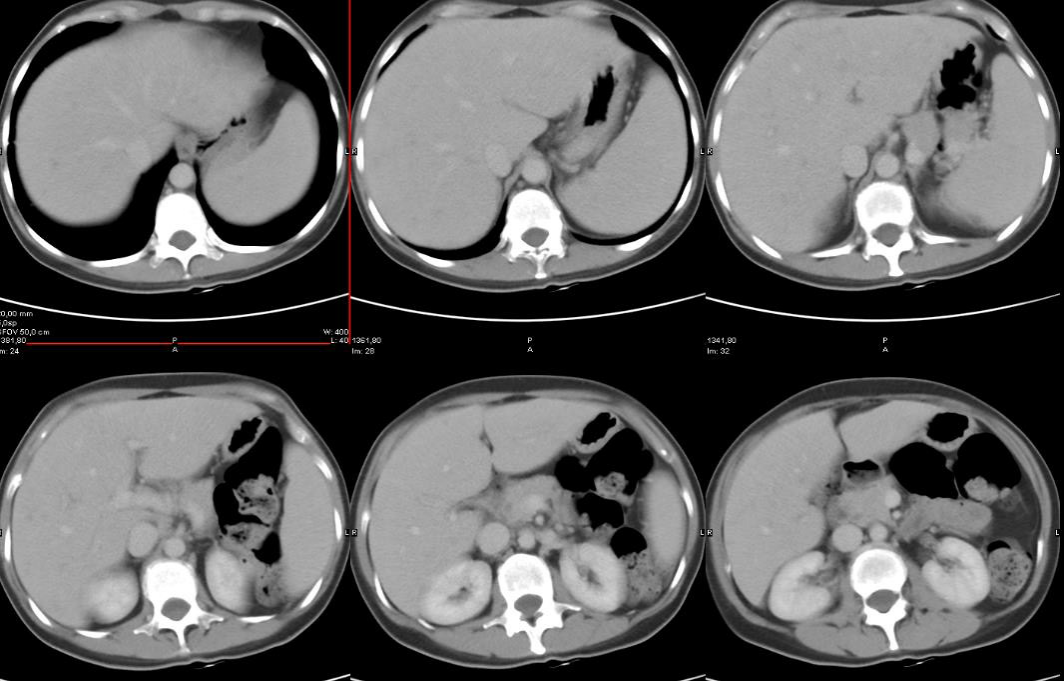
Anterior axial abdominal CT images (portal phase) show the presence of multiple hypodense nodules with a diameter between 5 mm and 1 mm, inhomogeneous enhancement, and ill-defined profiles
